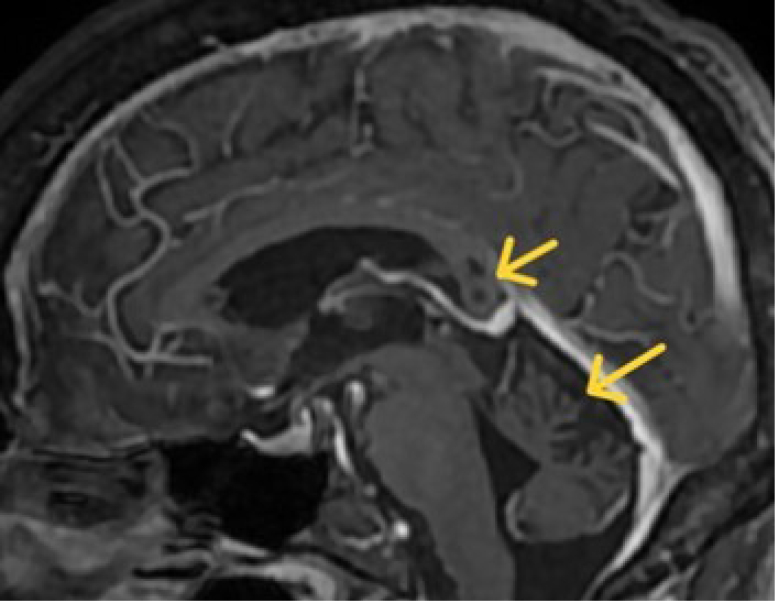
Brain magnetic resonance imaging sagittal T1 sequence, post-contrast, showing hypointensity of the splenium lesion and cerebellar vermis atrophy, no enhancement by the contrast.
